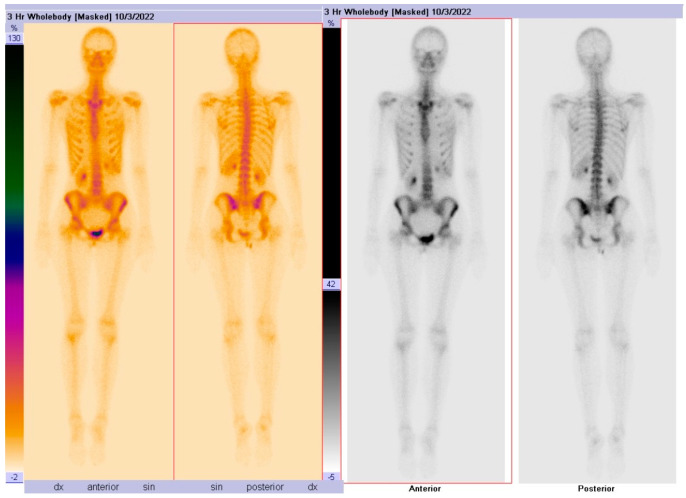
A static skeletal scintigraphy of the pelvic bones, which did not show conclusive evidence of pathological changes in the bones. No signs of osteoblastic lesions.
